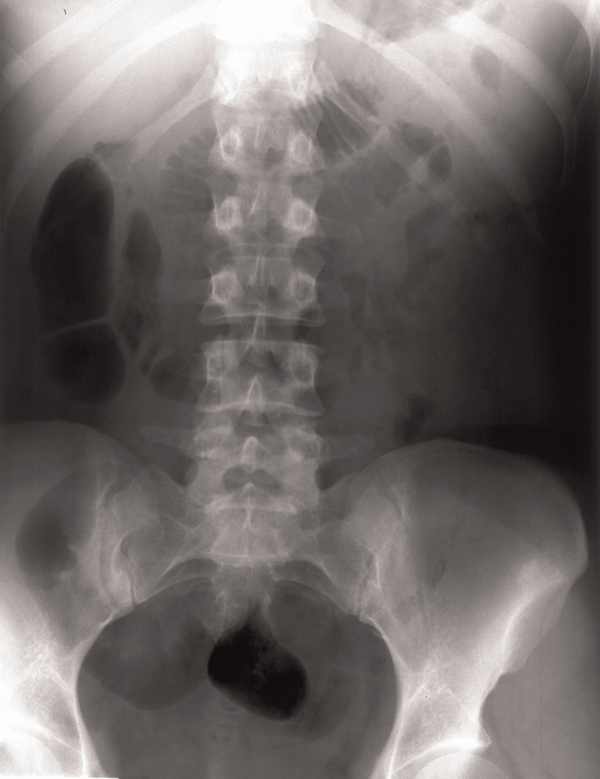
The kidney-ureter-bladder X-ray: no abnormal findings.
